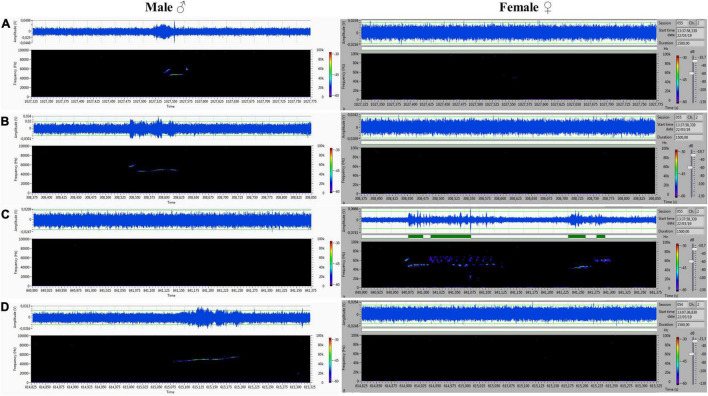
(A–D) Another set of ultrasounds with split type, upward, and step down on the male’s side, and frequency-modulated trills on the female’s side.
